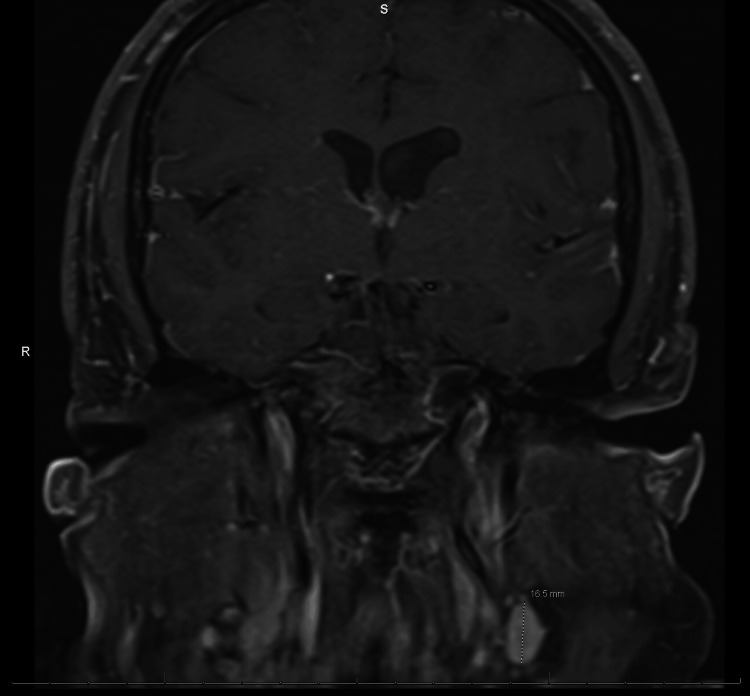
MRI brain and orbits with contrast demonstrating no abnormal enhancement or intracranial mass. Postcontrast sequences show preserved optic nerve sheath complexes and normal pituitary morphology without enhancement of the brain, optic nerves, or orbital structures.
